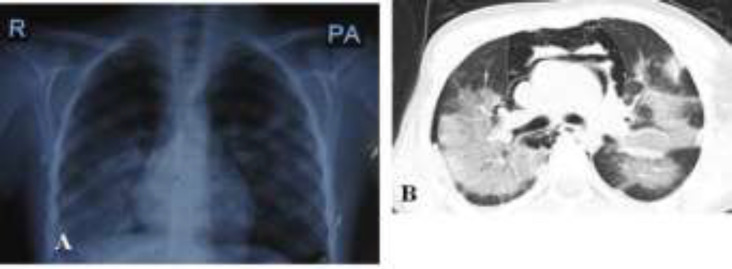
A, B CXR and lung CT findings: Multilober GGO and consolidation
